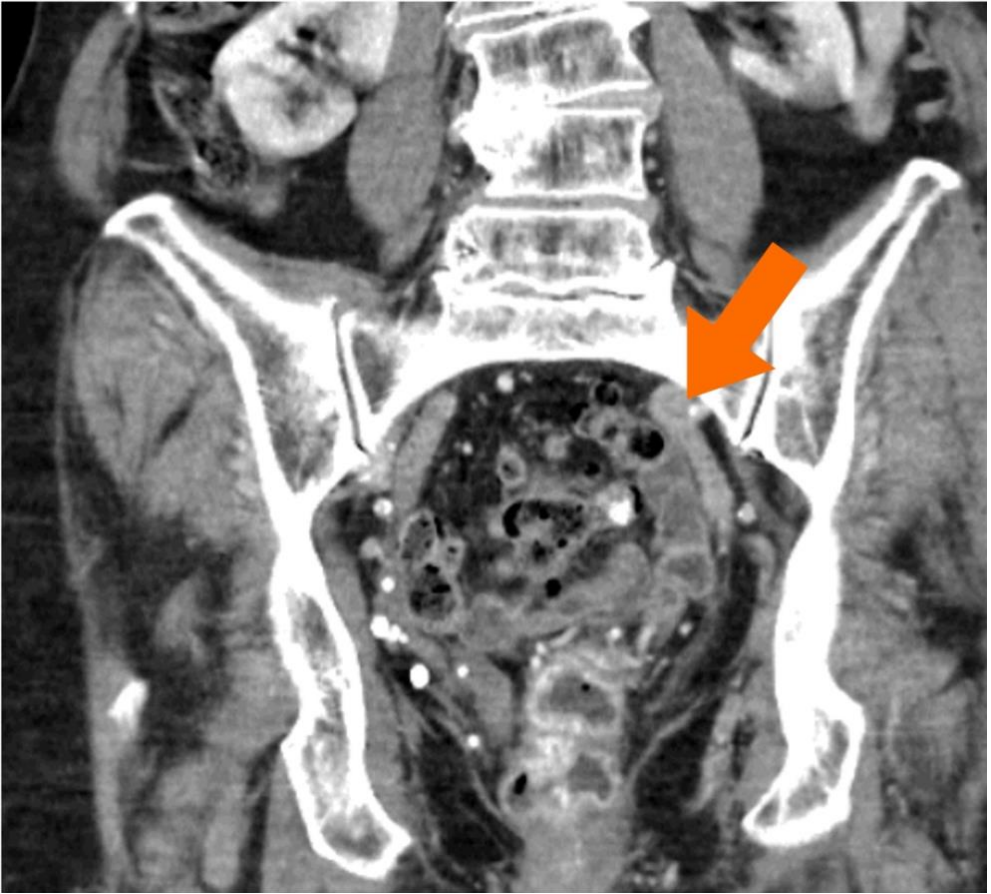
Radiologic imaging prior to surgery. The coronary view of the CT scan shows the crowed small pelvis filled with filled colon windings (orange arrow).
